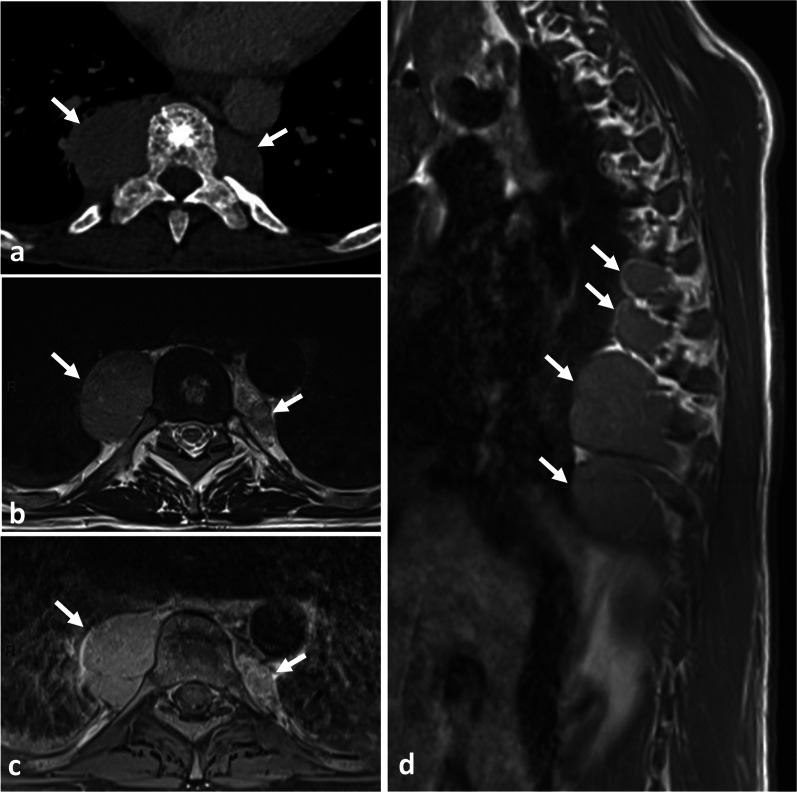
Extramedullary haematopoiesis* in a 25-year-old male with sickle cell disease. Contrast-enhanced axial CT scan reconstruction in the bone window (a), axial T2WI (b) and axial contrast-enhanced T1WI (c) show well-defined, bilateral hypaxial masses (arrows). Sagittal T1WI demonstrates multiple hypaxial masses (arrows) (d). Axial CT scan reconstruction (a) shows widened ribs and osteosclerosis of the vertebra
